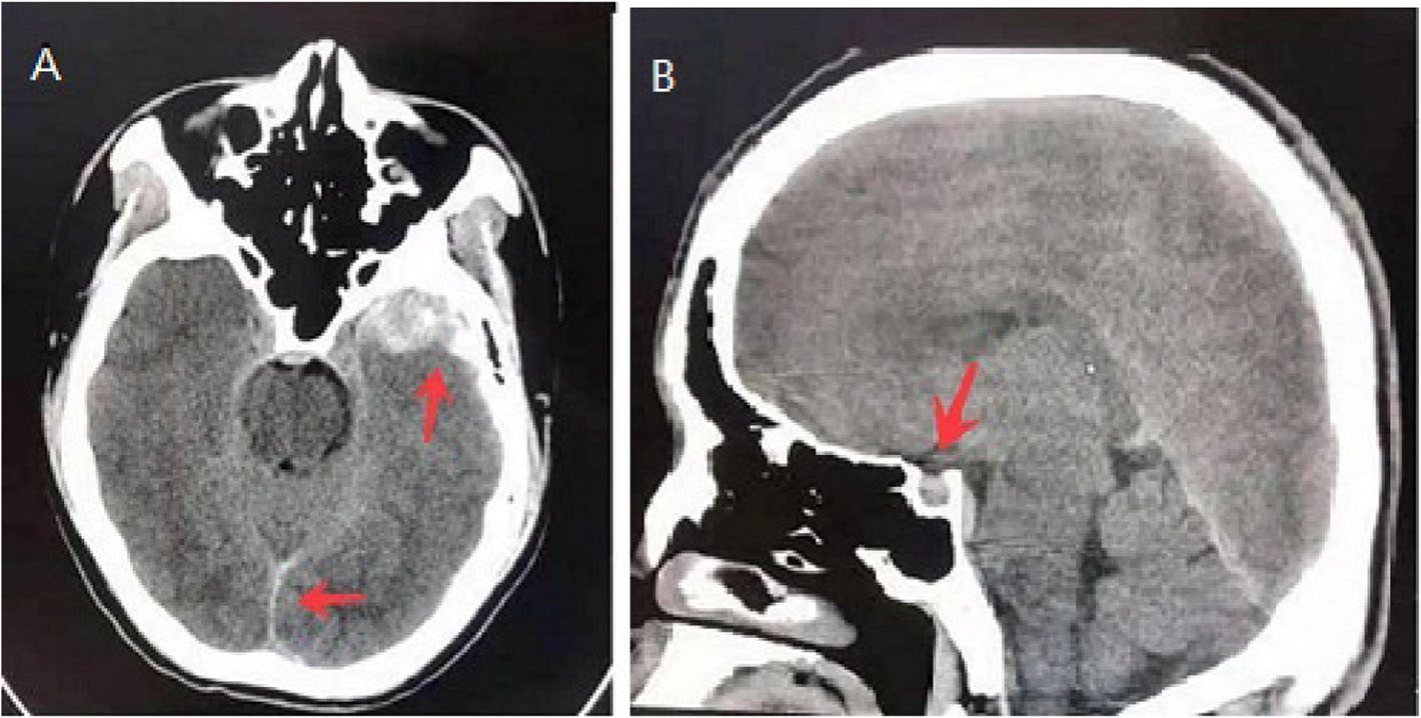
Pituitary CT at trauma 6 months ago showing subarachnoid and left subdural haemorrhage (Fig. 1a) and pituitary microadenoma (Fig. 1b)
